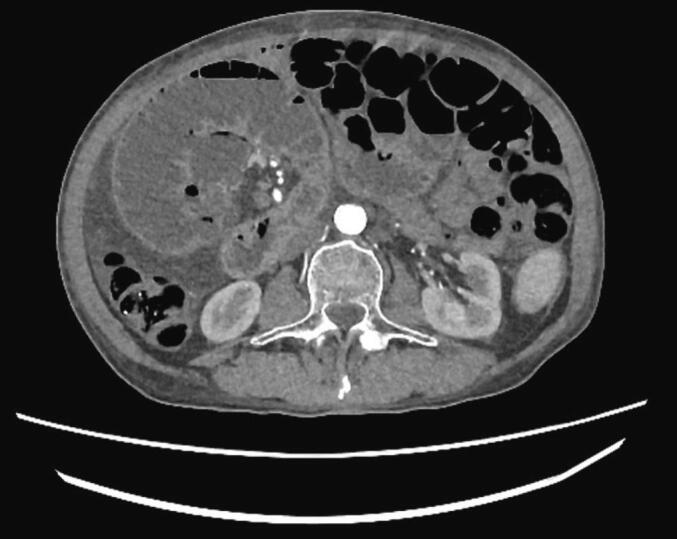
The first loop of the jejunum rotation showed on abdominal computed tomography (CT).
